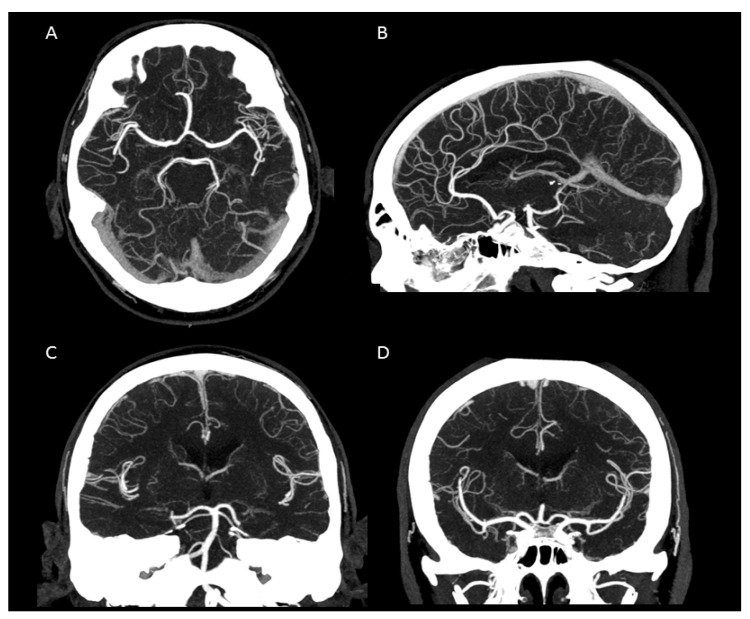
Maximum intensity projection of an intracranial UHR-CTA. (A) axial plane. (B) sagittal plane. (C,D) coronal plane. Note the clear traceability/visualization of the circle of Willis and even the small peripheral vessel branches.
